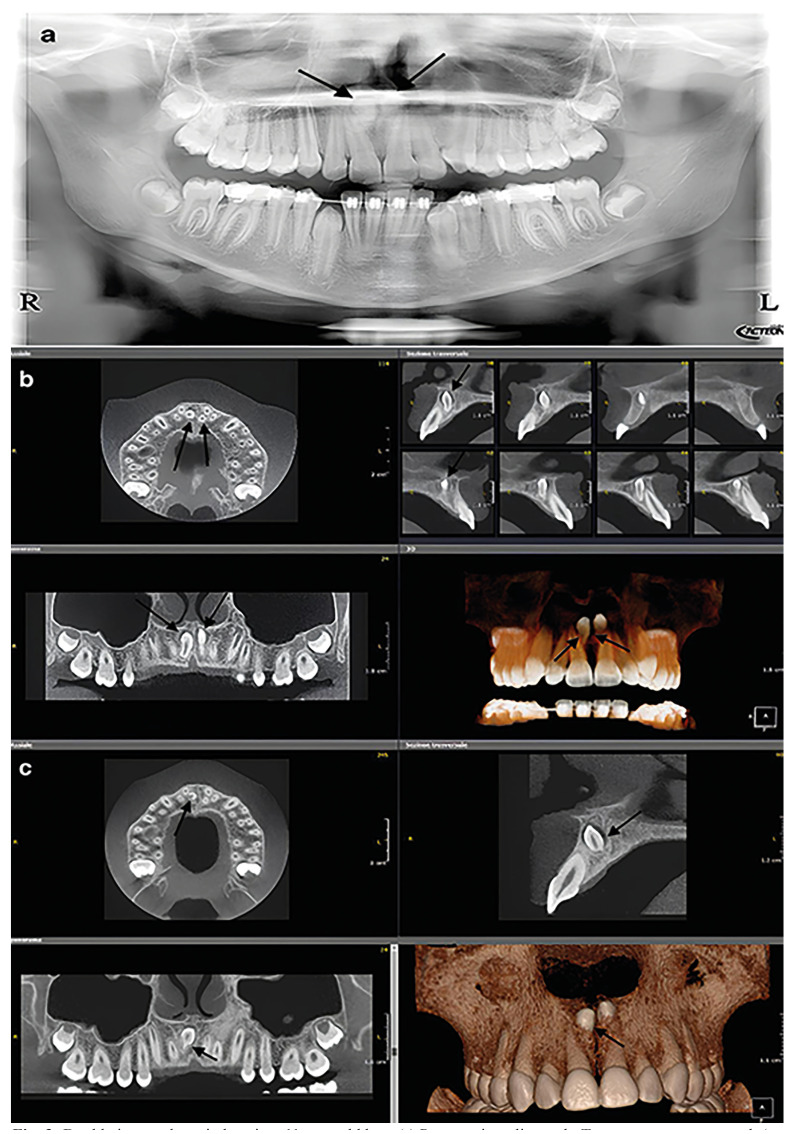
Double inverted mesiodens in a 11-year-old boy. (a) Panoramic radiograph. Two supernumerary teeth (arrows), partially overimposed to the hard palate, are seen between 11 and 21, whose roots are obliquely angled and distally displaced. (b) CBCT. The conoid shape of the two inverted mesiodens (arrows) is appreciable. Moreover, the palatal position, the relation with the nasopalatine canal and the contiguity of the 21 crown with the above nasal floor are demonstrated. (c) CBCT, 1-year follow-up. The right inverted mesiodens shows mild root resorption (arrow) compared to the initial CBCT (R=right; L=left).
